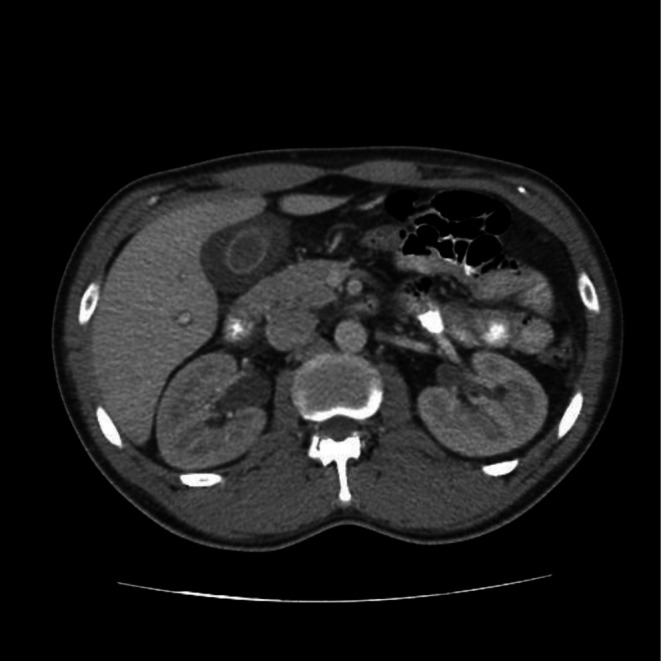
Axial post‐contrast abdominal CT scan showing pericholecystic edema surrounding the gallbladder without evidence of gallstones or significant gallbladder distension.
